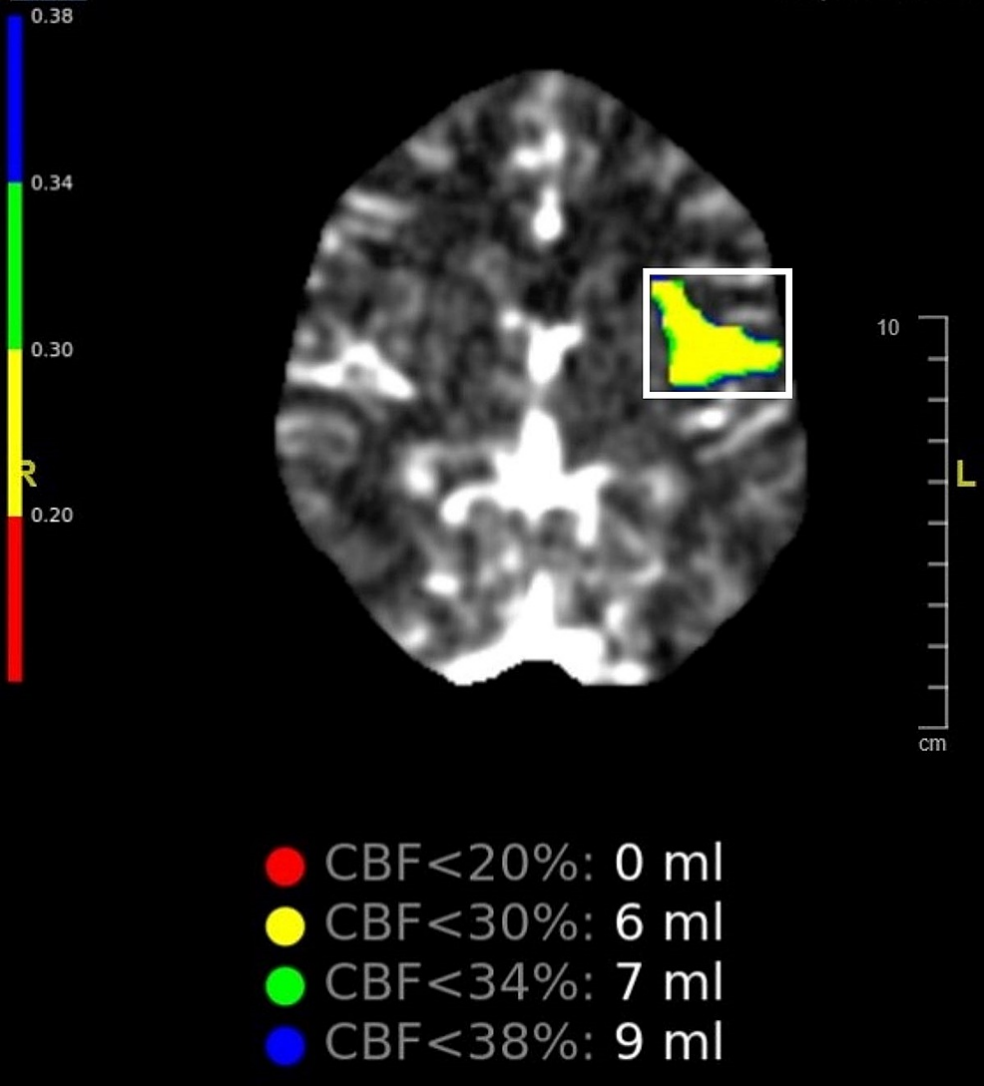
CT Angiography of the brain indicates area of relative perfusion deficits in the left middle cerebral artery (MCA) territory Cerebral blood flow of left MCA territory suggestive of 6mL of core infarct with less than 30% volume, shown in yellow color within a white box.
